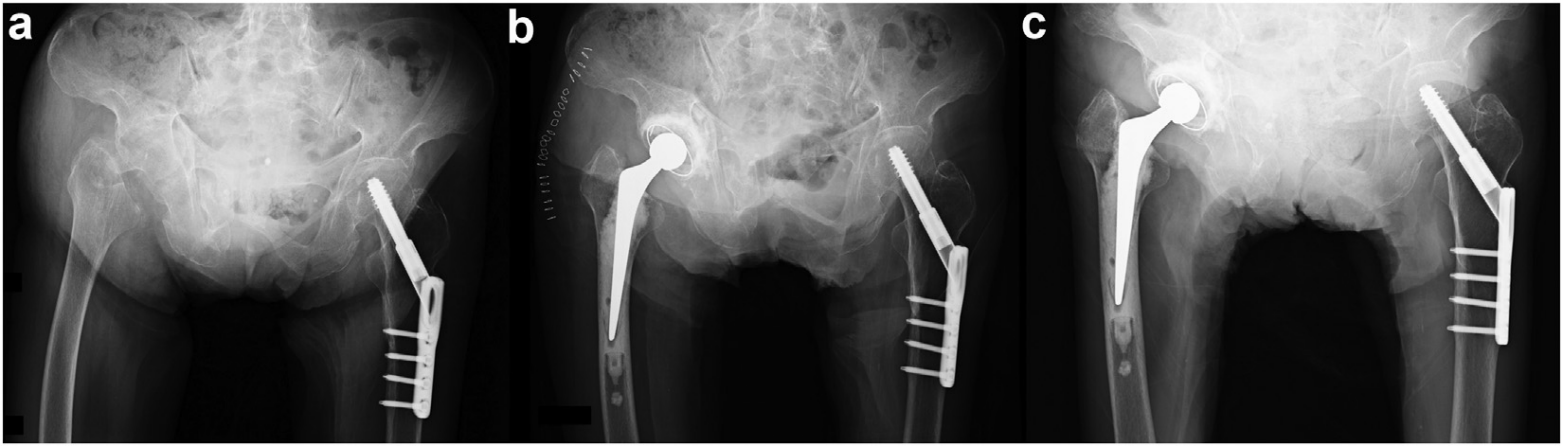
A case presentation (case 2). (a) Radiographic imaging at the patient’s first visit to our hospital showed a comminuted acetabular fracture with quadrilateral surface destruction as well as a femoral neck fracture. Good callus formation was evident on the acetabulum. (b) Radiographic images after late THA with IBG 2 months after the initial trauma. (d) Radiographic image at the 6-month postoperative follow-up showed no component loosening or any subsidence of the acetabular cemented cup. The patient died of pneumonia 2 weeks after our 6-month follow-up.
